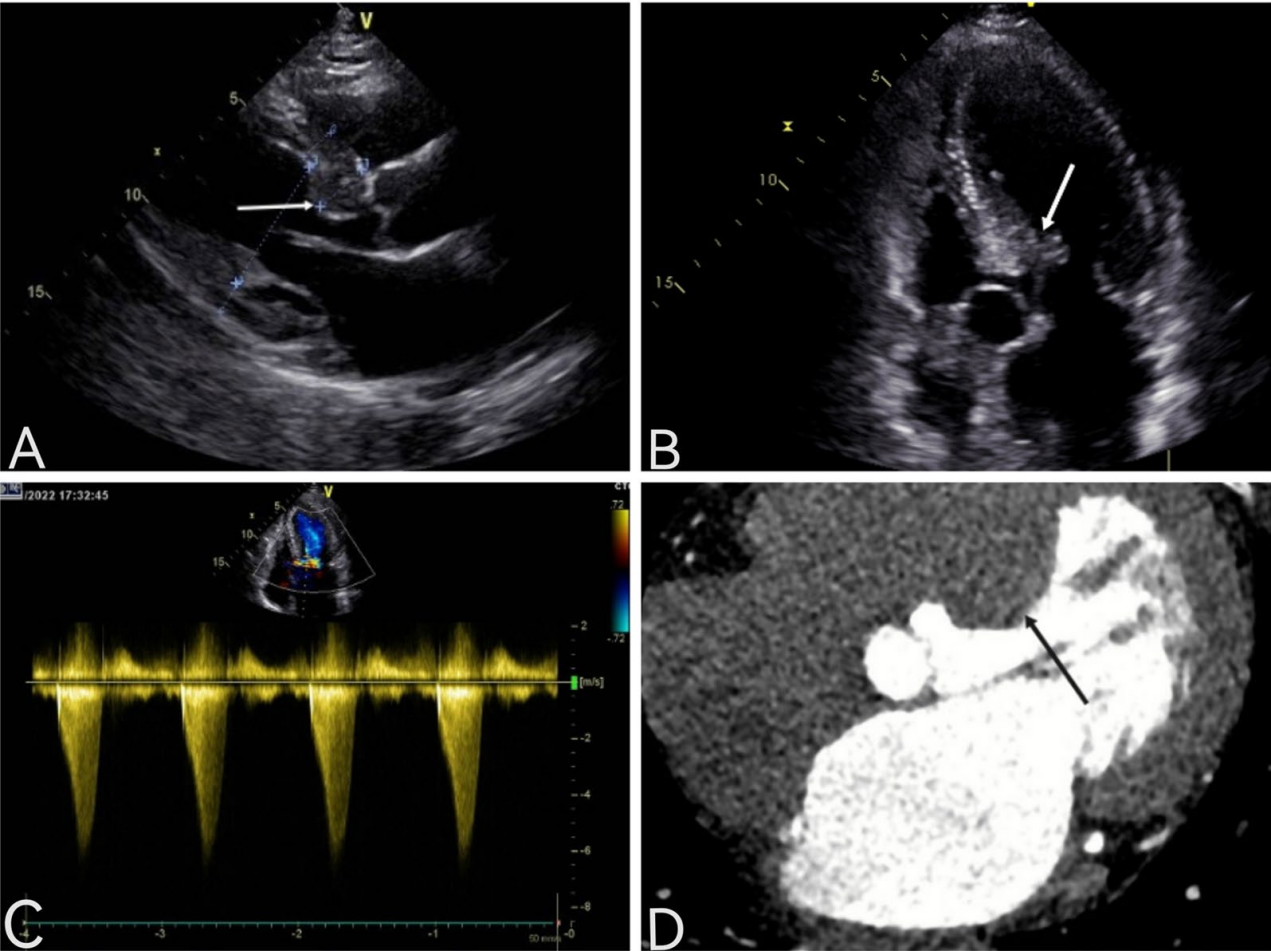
Echocardiography preoperativelythe basal part of the interventricular septum (IVS) was thickened (A, arrow).SAM was obvious (B, arrow).Systolic blood flow image in a specific direction from the left ventricle to the aorta (C, arrow).Coronary CTA showing the hypertrophic myocardium clearly protrudes into the left ventricular outflow tract (LVOT) (D, arrow)
